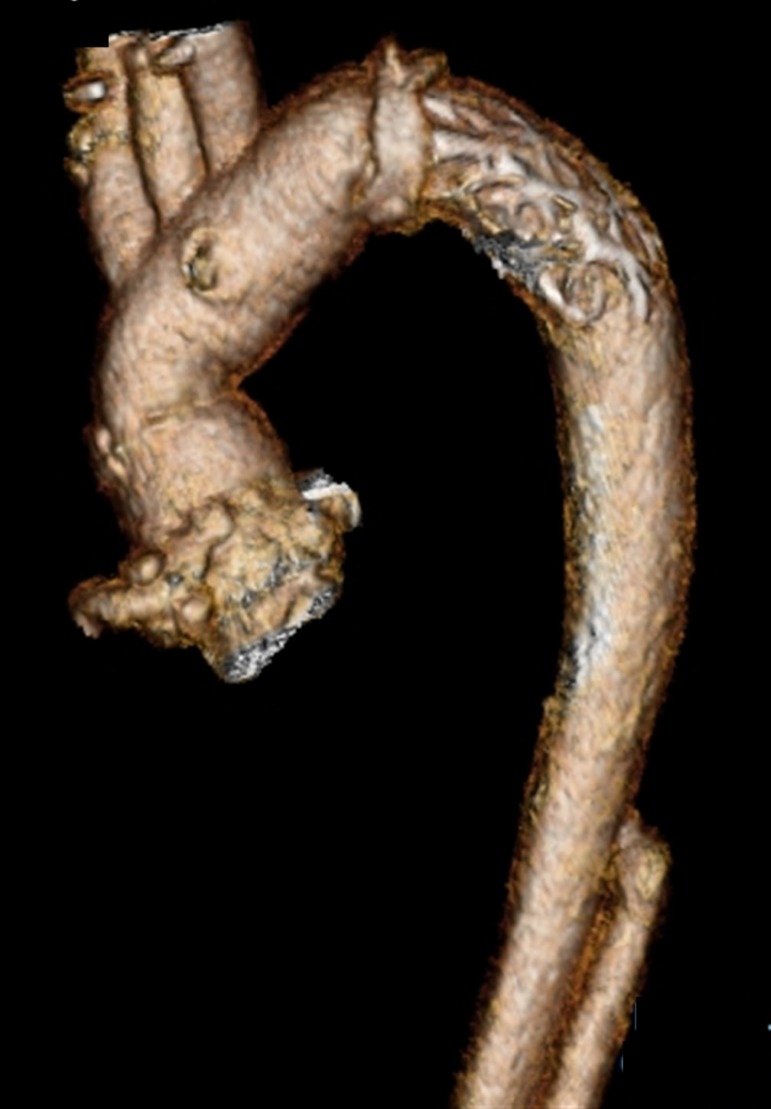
Postoperative computed tomography image Repair of the dissected aorta is
